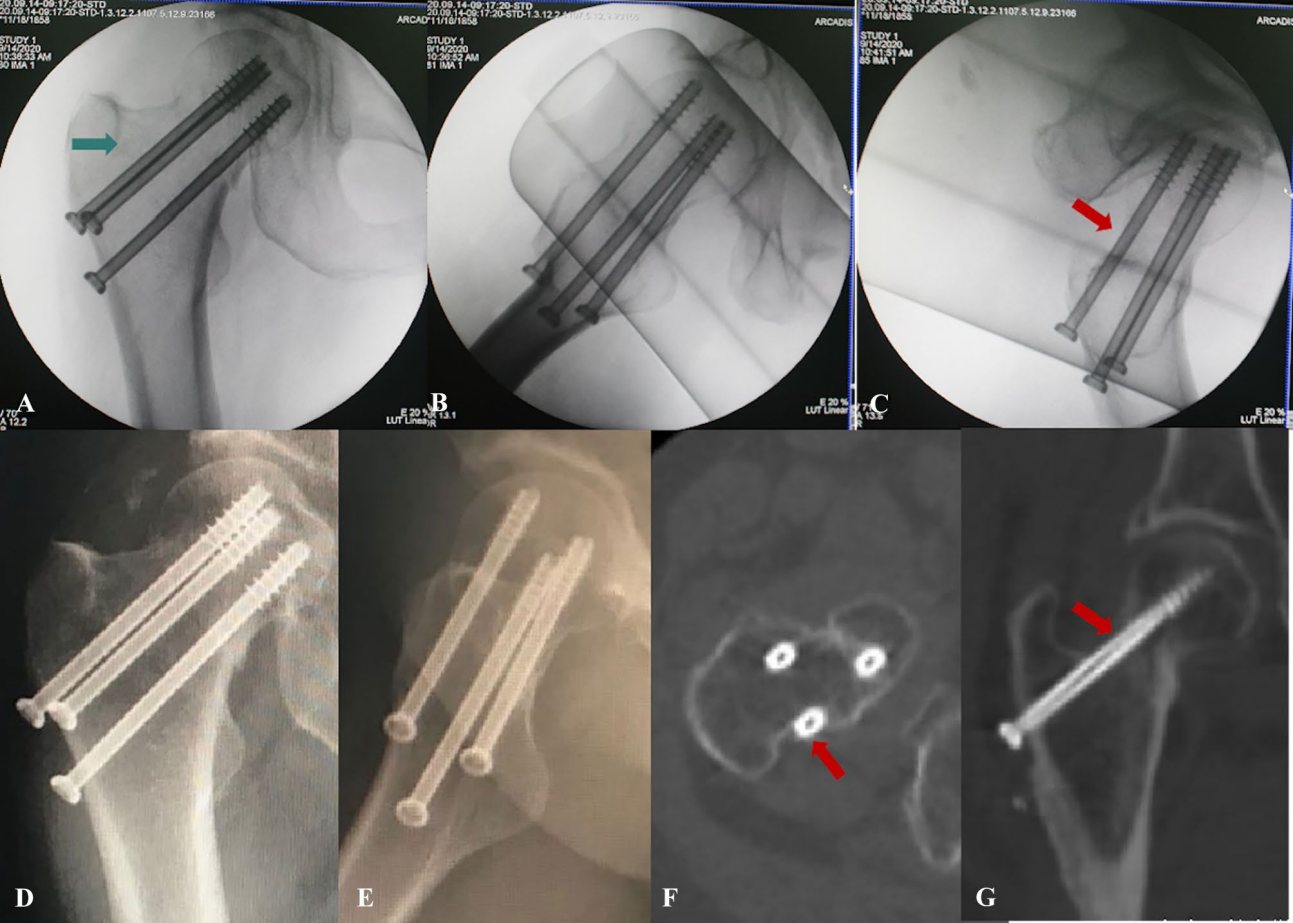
The posterosuperior femoral neck screw placed caudal to the inferior margin of the piriformis fossa in–out–in. The green arrow represents the piriformis fossa radiographic landmark. The red arrow represents the posterosuperior femoral neck screw IOI. (A,B) Intra-operative the AP and lateral views showed all screws were contained within the femoral neck. (C) Intra-operative the tangential view showed the posterosuperior screw IOI. (D,E) Postoperative plain radiographs showed all screws were contained within the femoral neck. (F,G) Postoperative CT showed the posterosuperior femoral neck screw IOI.
